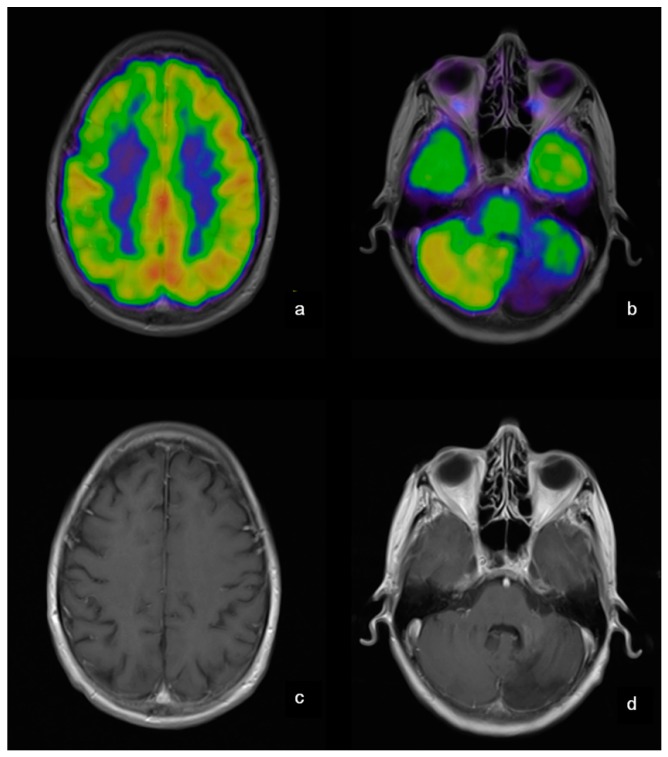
Patient no. 25 suffered from a cerebellar hemorrhage and developed a hypometabolic area corresponding to tissue loss in the left cerebellar hemisphere (b + d) in addition to a cerebellar cortical diaschisis (a + b). (a) Cortex (MRI fusioned with PET)–decreased activity in the right hemisphere; (b) Cerebellum (MRI fusioned with PET)—decreased activity in the left cerebellar hemisphere; (c) Cortex (MRI)—no structural changes; (d) Cerebellum (MRI)—sequelae after hemorrhage. This research was originally published in Reference [30].
